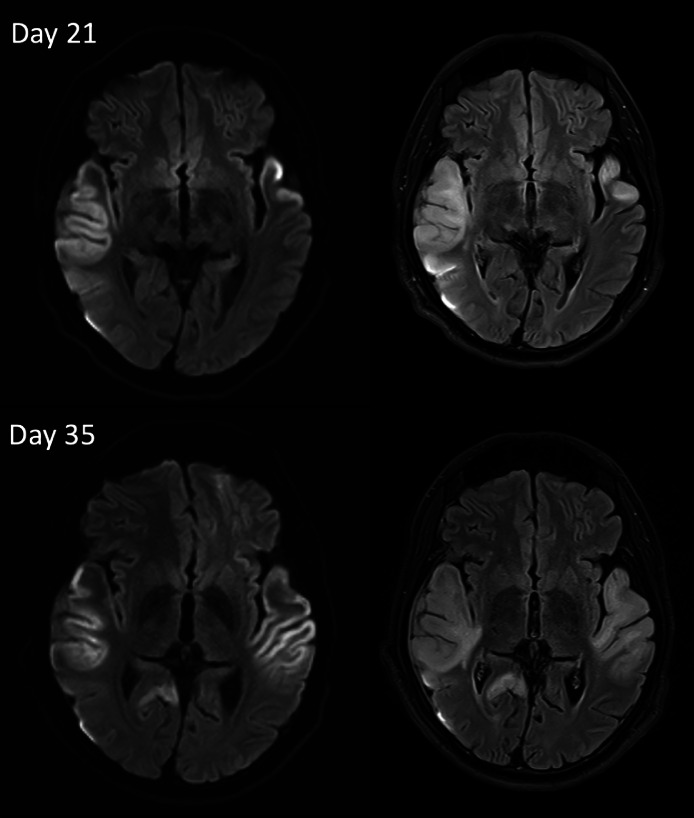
Diffusion restriction and FLAIR images from Day 21 and Day 35 show worsening cortical based diffusion restriction in the left temporal lobe in combination with subcortical edema and component of new cortical based diffusion restriction in the right posterior temporal occipital PCA territory.
